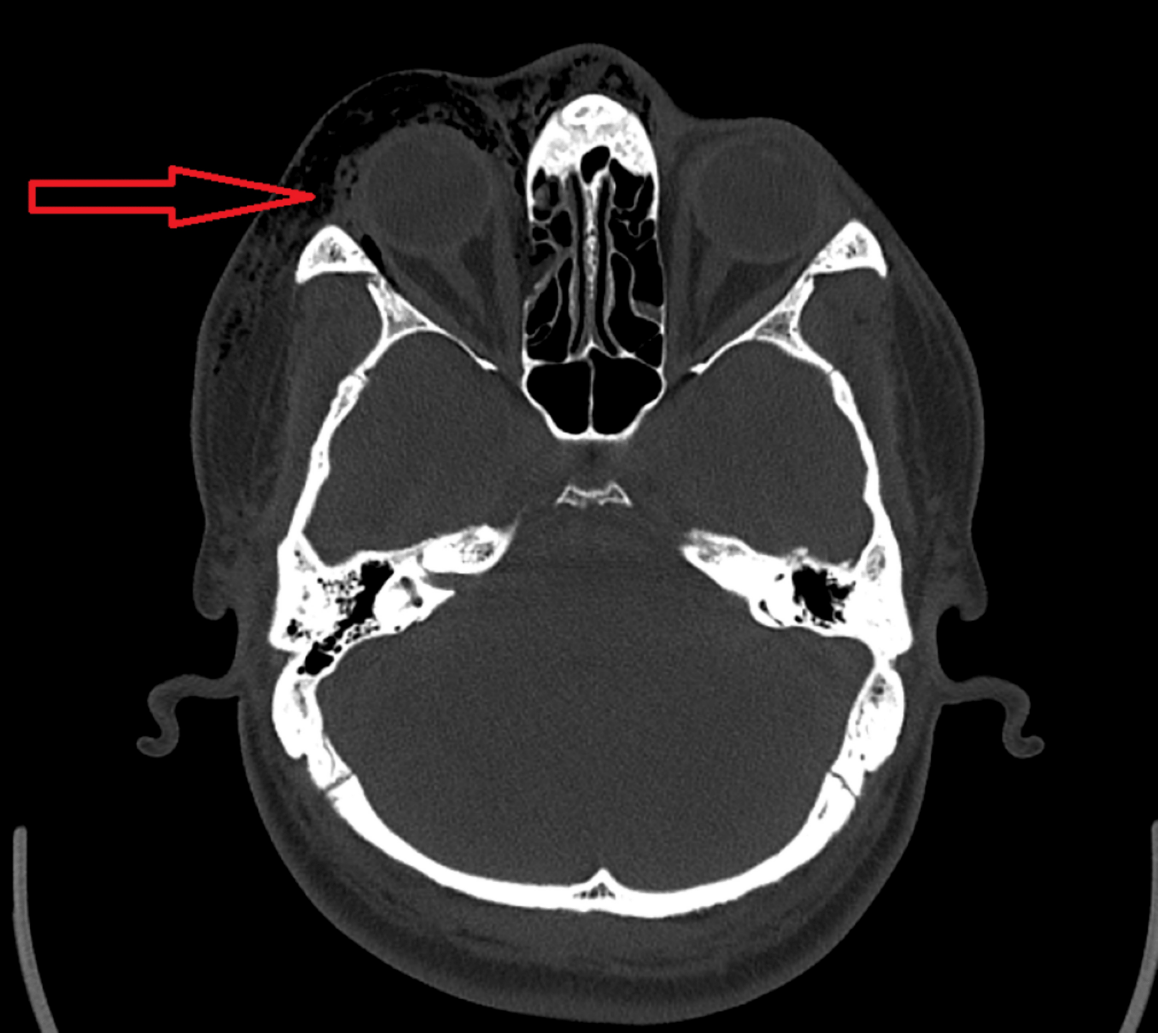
Axial CT view demonstrating right orbital subcutaneous emphysema as pointed out by the red arrow
